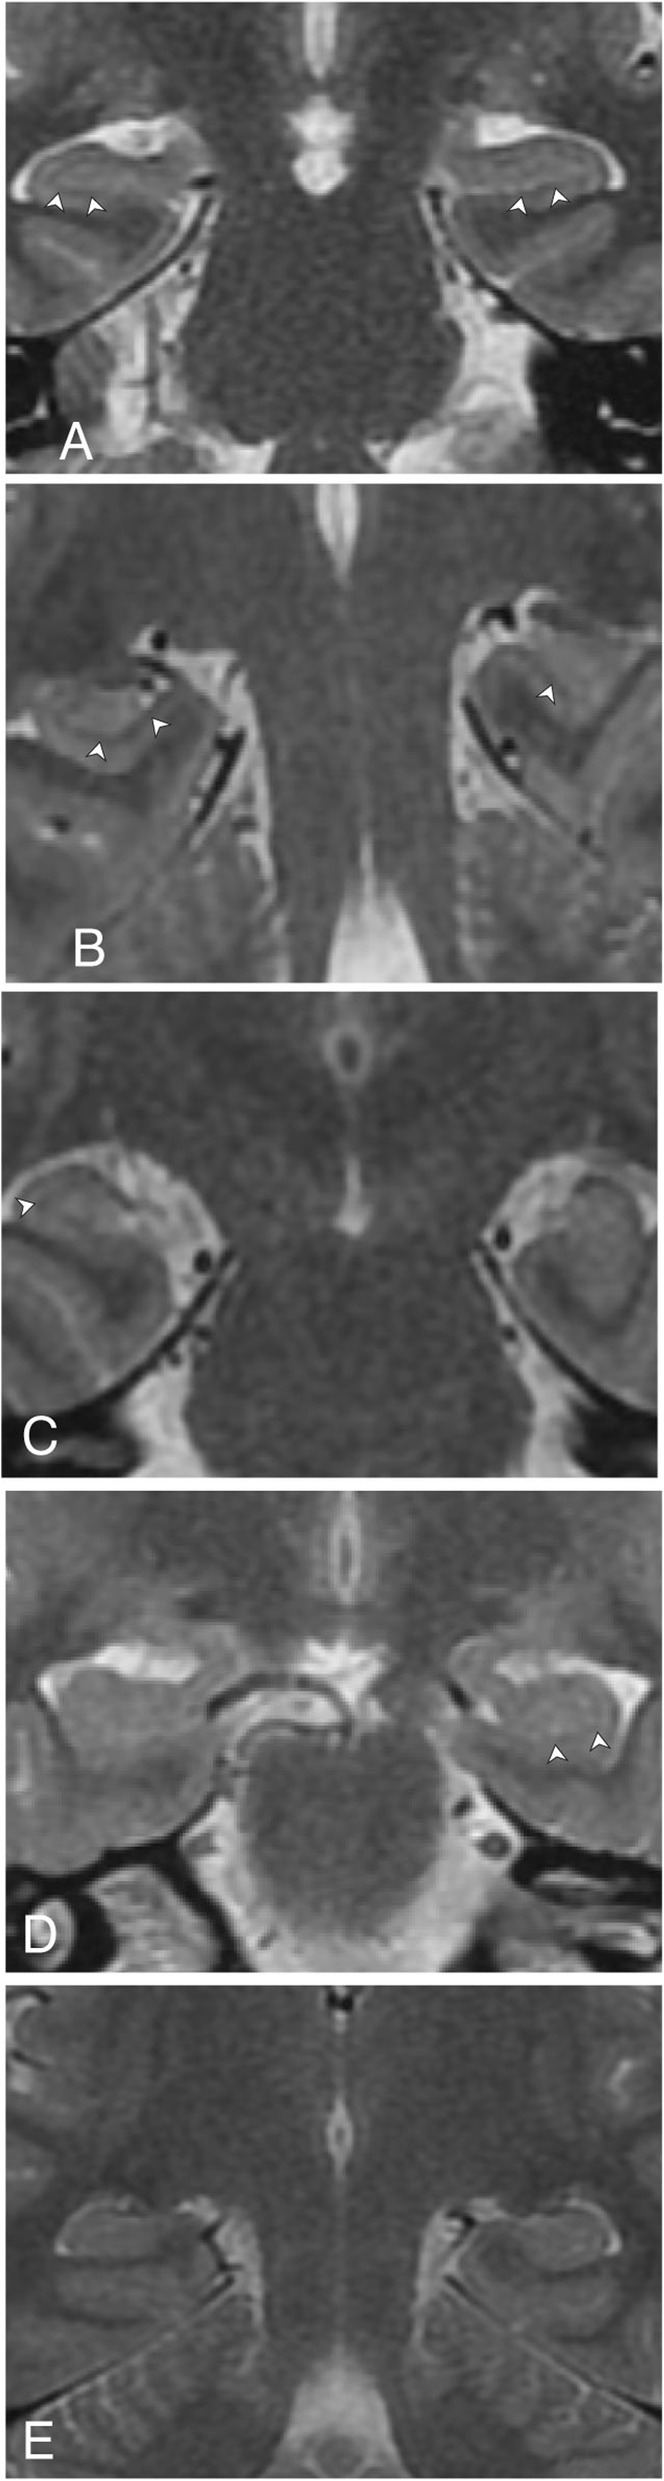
a–e High-resolution coronal T2-weighted images with magnification. Arrowheads indicate hippocampal striations. a Normal hippocampus: hippocampal striations seen on both right and left sides. Symmetry of semicircular canals can be seen along bottom corners of image on either side. b Left PLHS: hippocampal striation seen better on the right side. On the left side, it is seen only in medial aspect of hippocampus, not in its lateral aspect. c Left PLHS: hippocampal striations seen on the right side, not on the left. d Right PLHS: hippocampal striation seen faintly on the left; not on the right. e Bilateral PLHS: hippocampal striation not well seen on either side
